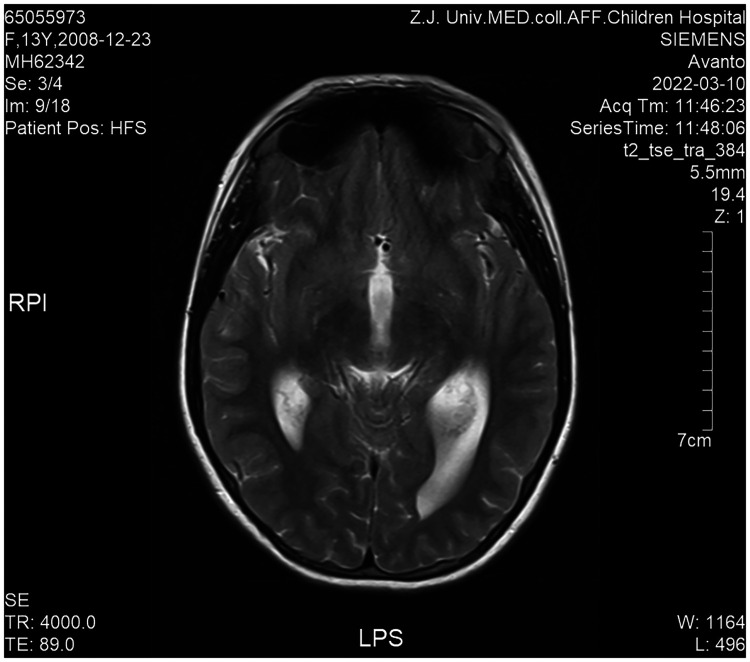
Brain MRI (sagittal position).
